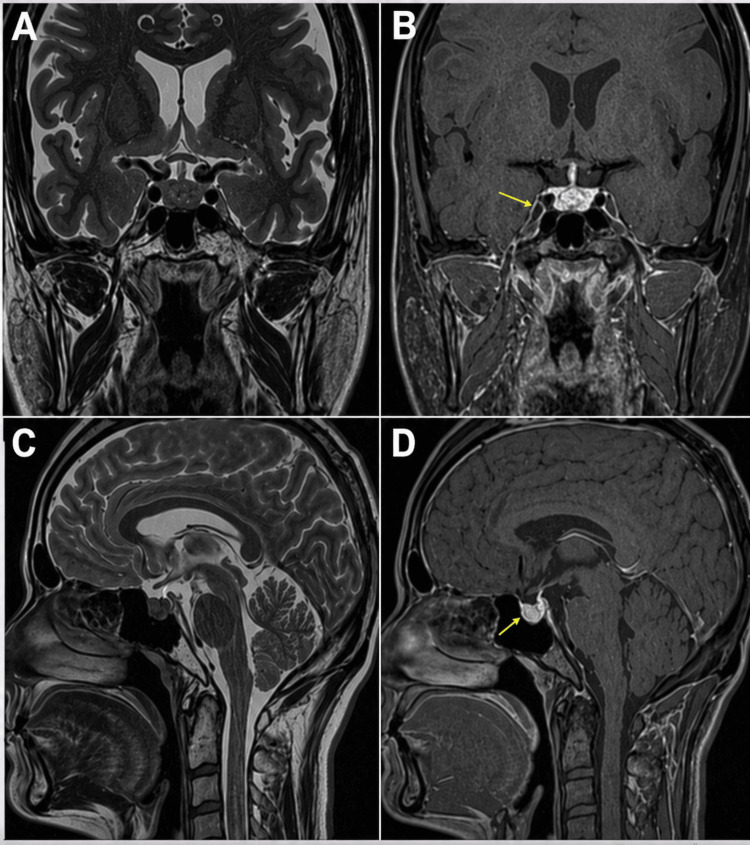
MRI of the brain with pituitary protocol demonstrating immune checkpoint inhibitor–related hypophysitis A. Coronal T2-weighted MRI demonstrating normal appearance of the pituitary gland and stalk. B. Contrast-enhanced coronal T1-weighted MRI demonstrating homogeneous enhancement and expansion of the pituitary gland and stalk (yellow arrow), consistent with hypophysitis. C. Sagittal T2-weighted MRI demonstrating normal size and morphology of the pituitary gland and stalk. D. Contrast-enhanced sagittal T1-weighted MRI showing diffuse enlargement of the pituitary gland with thickening and increased enhancement of the pituitary stalk (yellow arrow), measuring approximately 5 mm, consistent with hypophysitis. MRI, magnetic resonance imaging
